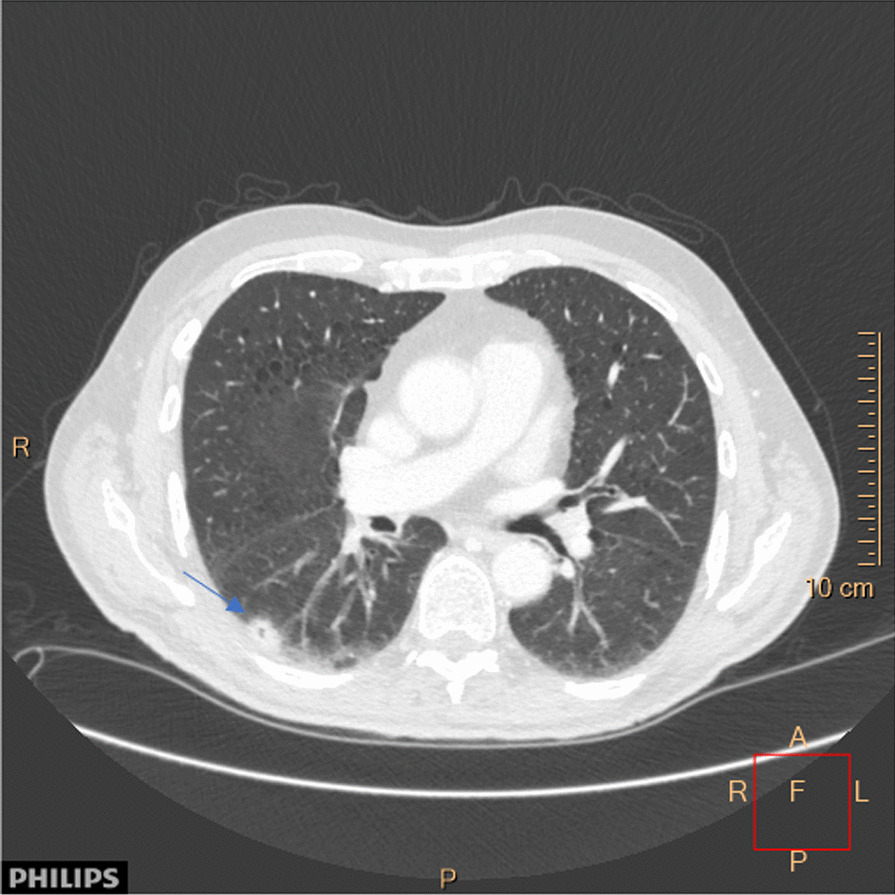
Computed tomography (CT) of the thorax axial view taken before the initiation of chemotherapy showing nodulus (blue arrow) in the lower lobe of right lung. (A: anterior; R: right; L: left; P: posterior, F: focus; cm: centimeter)
